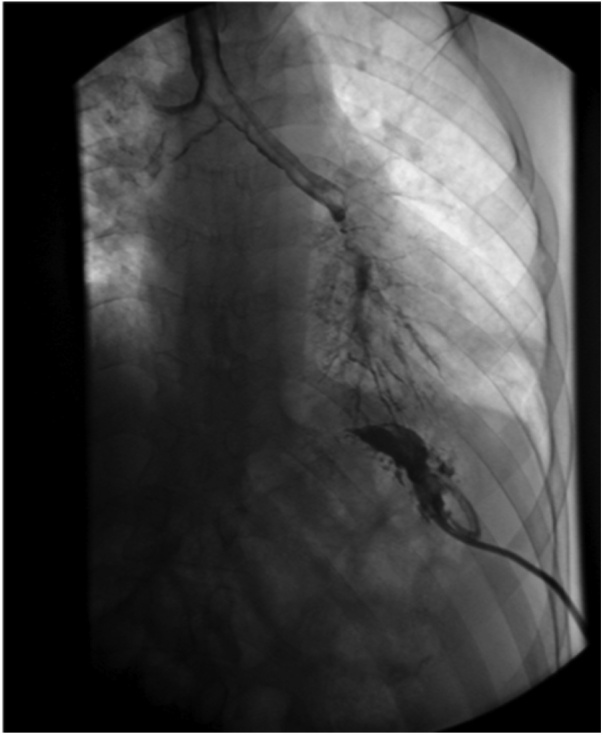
X-ray fluoro-fistulogram showing contrast from the left upper quadrant collection to be freely communicating with the left lower lobe bronchioles reaching into the left main bronchus and trachea.
